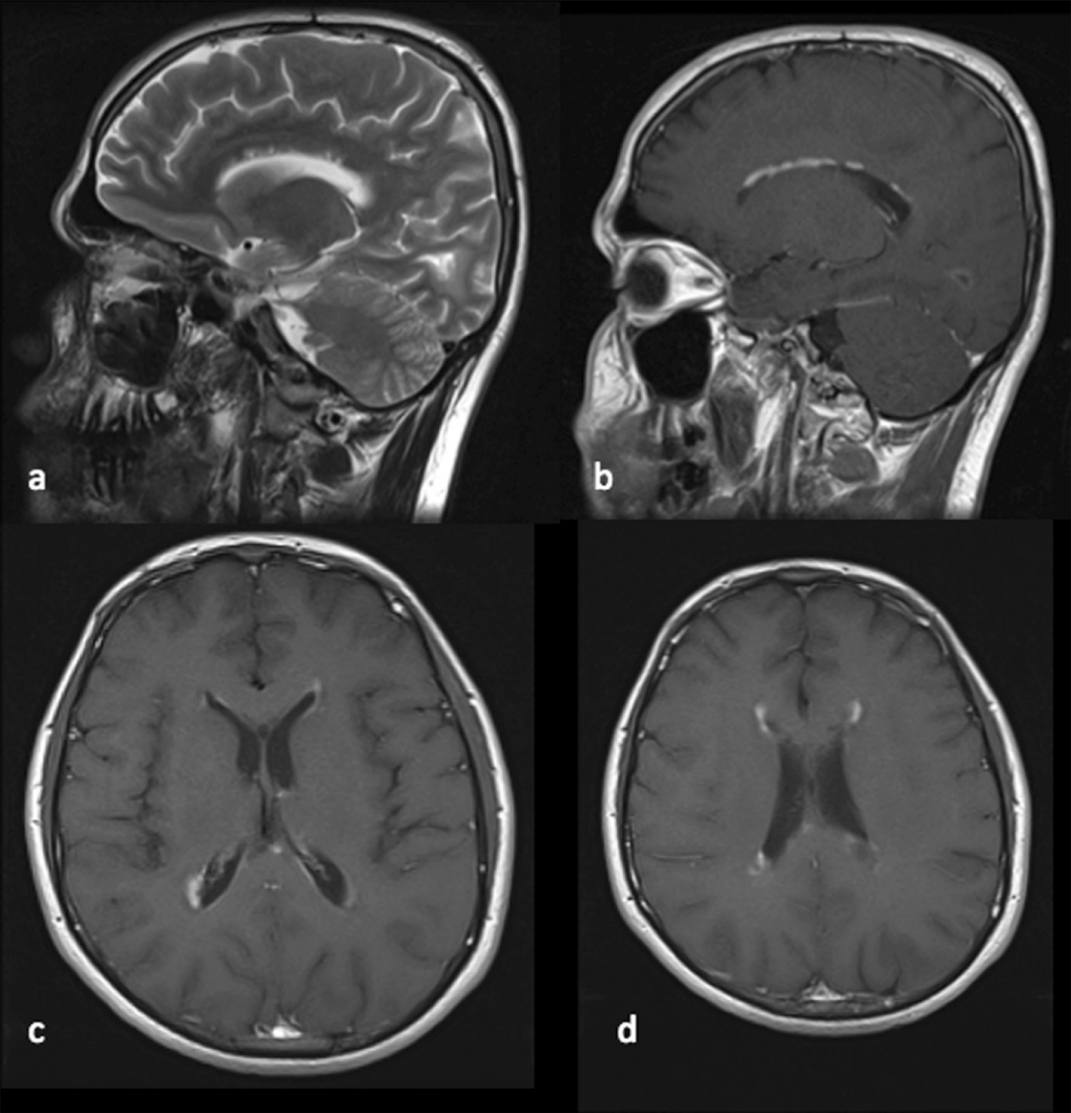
T2-weighted and T1-weighted images with contrast agent. a,b Images show involvement of the corpus callosum with linear enhancement. c,d Thin ependymal enhancement of lateral ventricular surfaces (pencil-thin ependymal enhancement)
